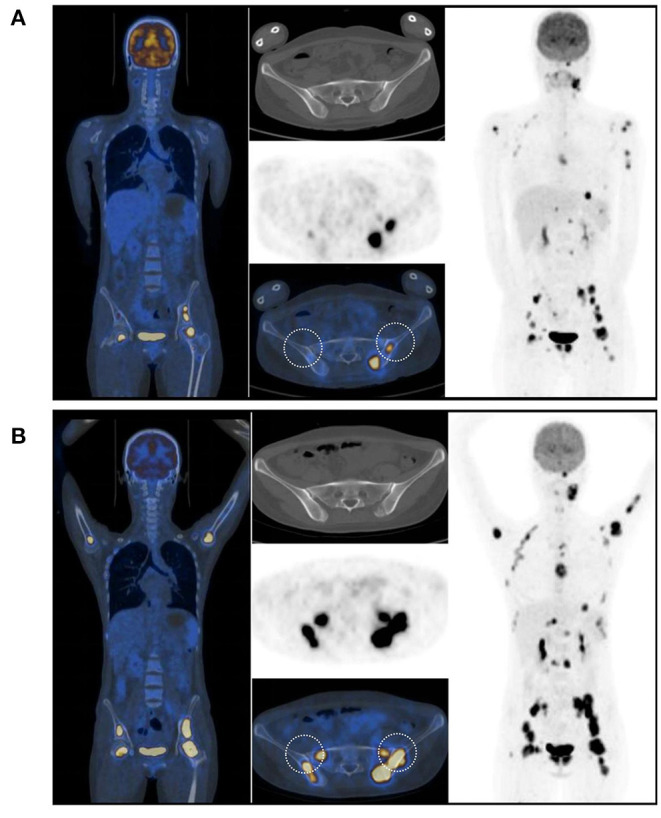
^18^F-FDG-PET/CT scans showed that asymmetrical hypermetabolic foci were noted across the bone marrow system. (A) on the day +143. (B) on the day +203. Both aspirations and biopsies were performed in the circle areas.
